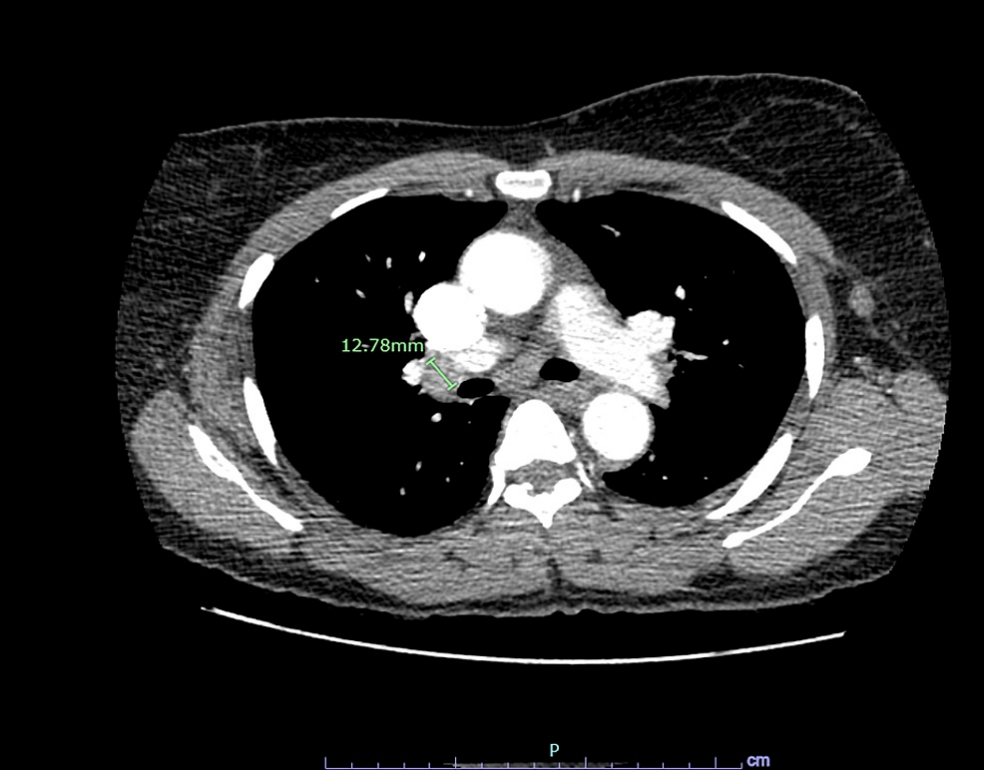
CTA showing right hilar lymphadenopathy of 1.3 cm CTA: computed tomography angiography
